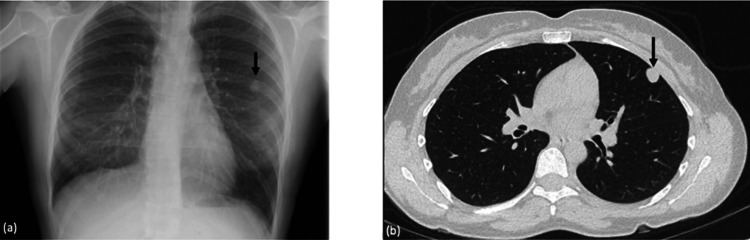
Initial imaging on investigation of a left-sided lung nodule (a) Chest x-ray showing a well-rounded nodule in the periphery of the left midzone measuring up to 1.5 cm. (b) CT thorax showing a solitary 14 mm subpleural nodule anteriorly in the lingula. Nodule is hyperdense (mean density 30-50 Hounsfield Unit (HU)) with probable cystic nature. Acute angulation with the costal surface suggests pulmonary rather than pleural origin.
